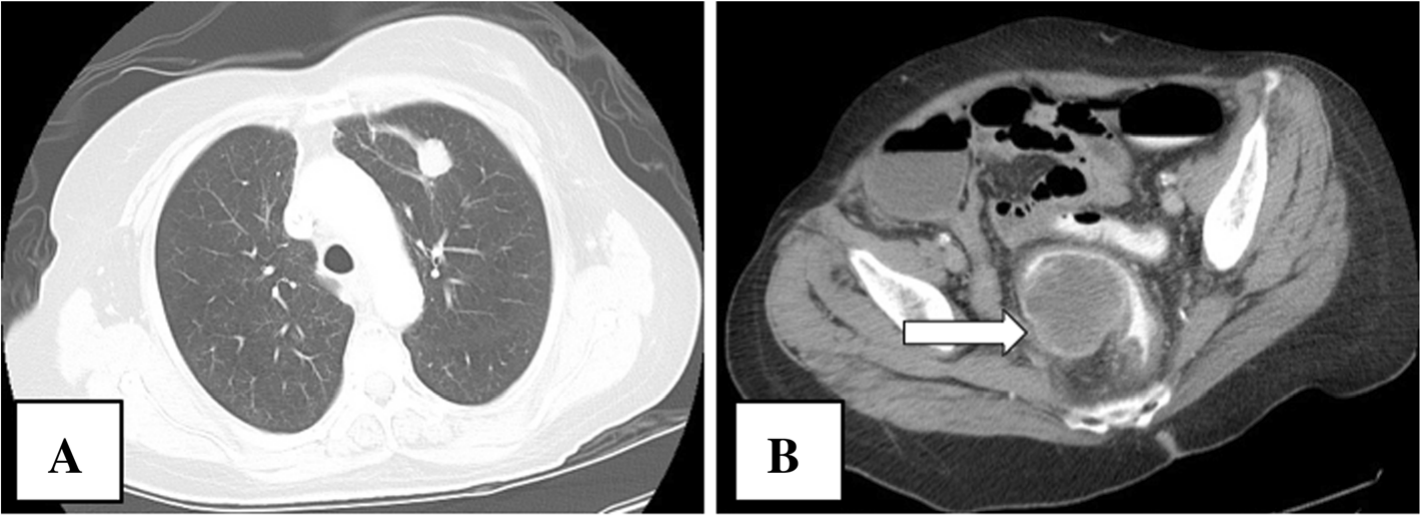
Imaging showed a tumor in the left lung field and sigmoid colon. a Chest CT revealed a residual soft tissue mass anteriorly within the left upper lobe measuring approximately 2.0 × 1.7 cm without appreciable adenopathy (not shown) consistent with the patient’s known history of lung cancer. b Abdomen and pelvic CT showed a soft tissue mass with approximate 5.0 × 4.7 cm dimensions within sigmoid colon (*arrow*) at 15 cm from the anal orifice. A sigmoid mass with extrinsic features and mucosal involvement can be seen contributing to marked narrowing of the sigmoid colon, but allowed contrast to pass through area of narrowing
